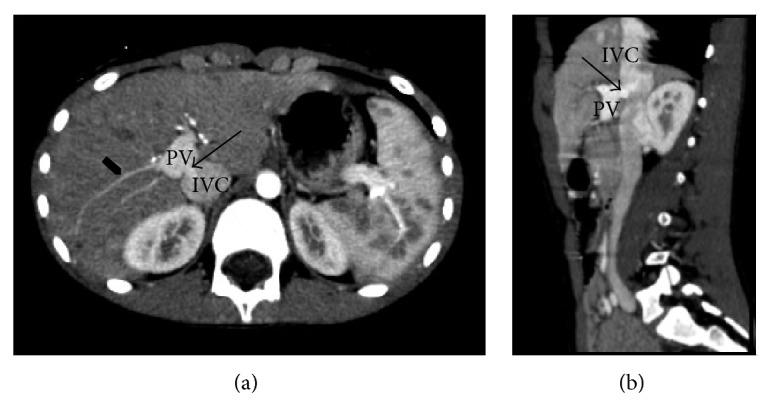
Contrast-enhanced CT images. Axial projection (a); sagittal-oblique projection (b). The arrows in (a) and (b) show a shunt between the posterior wall of the portal vein (PV), just before its intrahepatic hilar division, and the inferior cava vein (ICV); the intrahepatic portal branches appear reduced and filiform (arrowhead in (a)).
